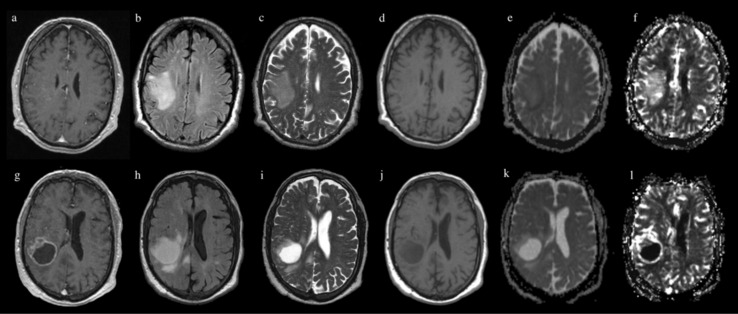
Examples of incorrectly predicted outcome (IDH mutation) from our CNN model. Images above show MPRAGE (a), FLAIR (b), T2 (c), T1 (d), ADC (e) and rCBV (f) sequences of a patient with IDH mutated GBM predicted as wild type (false negative). Images below show MPRAGE (g), FLAIR (h), T2 (i), T1 (j) ADC (k) and rCBV (l) sequences of a patient with IDH-wild-type GBM predicted as mutated (false positive).
